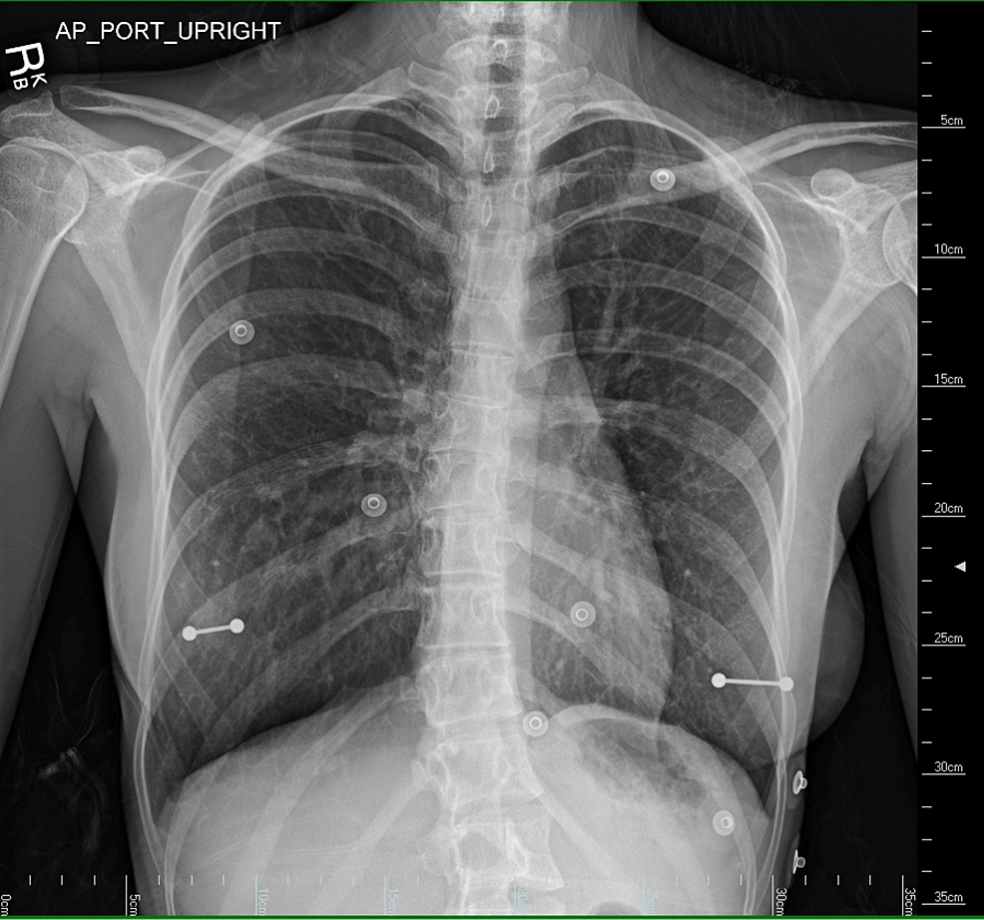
Chest radiograph of a 28-year-old woman showing subtle right lung hyperinflation
